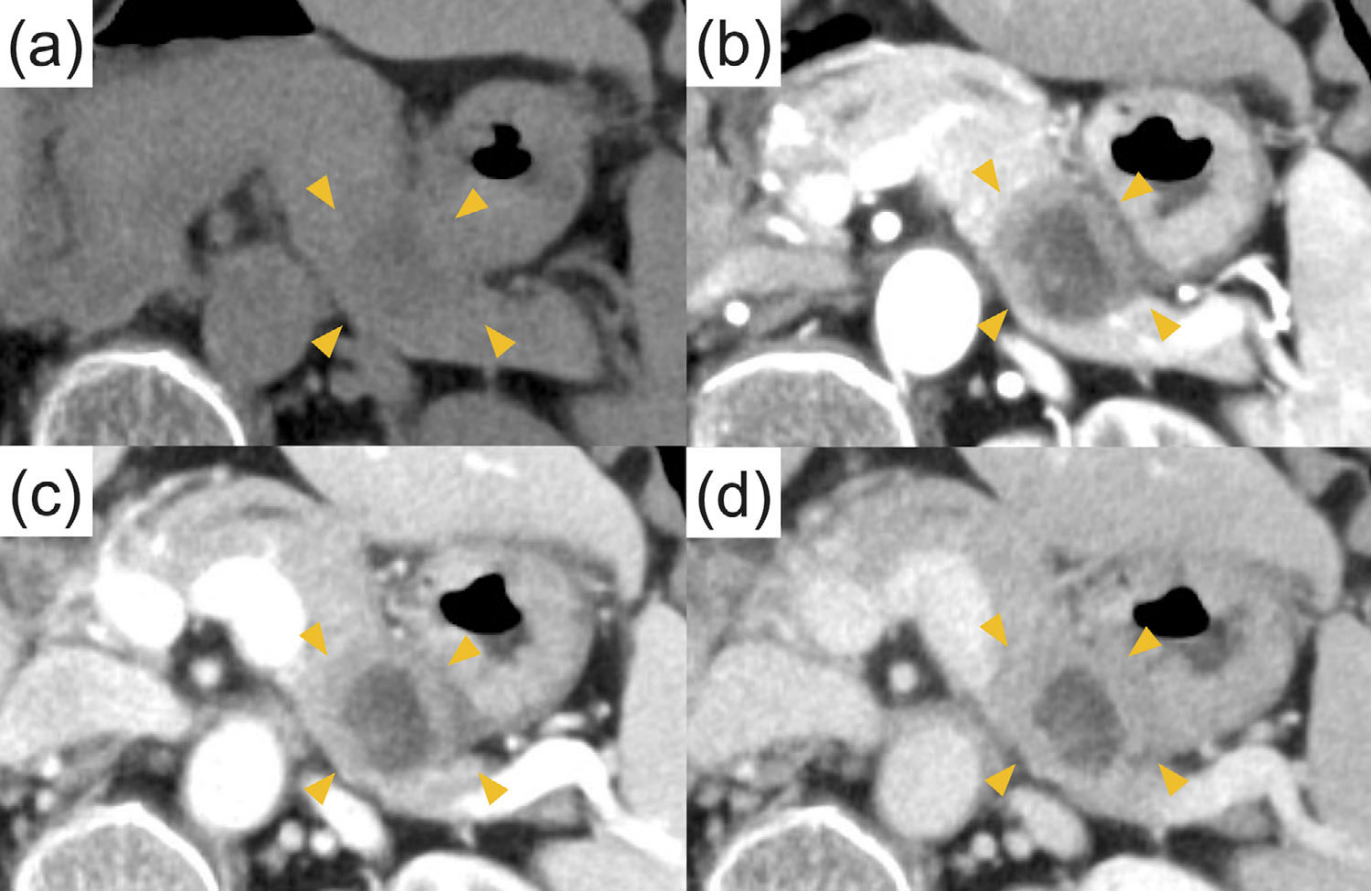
Abdominal computed tomography results: (a) noncontrast‐enhanced image; (b) late arterial phase; (c) portal phase; (d) delayed phase. A 20‐mm‐diameter hypovascular tumor is seen in the pancreatic tail (orange arrowheads). The lesion was round in shape, and a low‐density area suspected of necrosis or fluid retention was observed inside the lesion.
